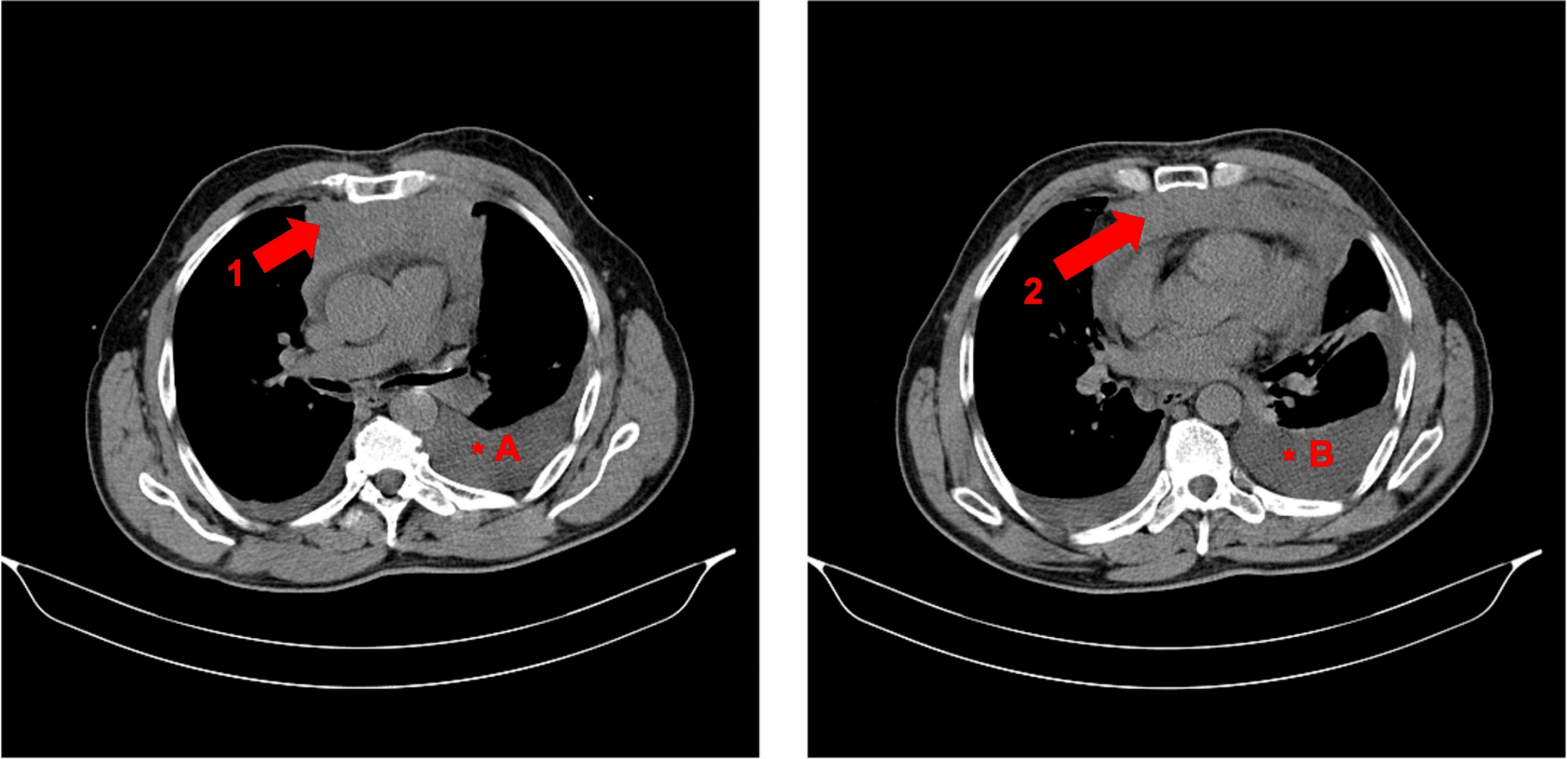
Axial chest CT scan (soft-tissue/mediastinal window) demonstrating a 12.4 × 3.2 cm anterior mediastinal mass. Red arrows (1) and (2) indicate the anterior mediastinal mass, while asterisks (*A and *B) denote pleural effusions. CT: computed tomography
